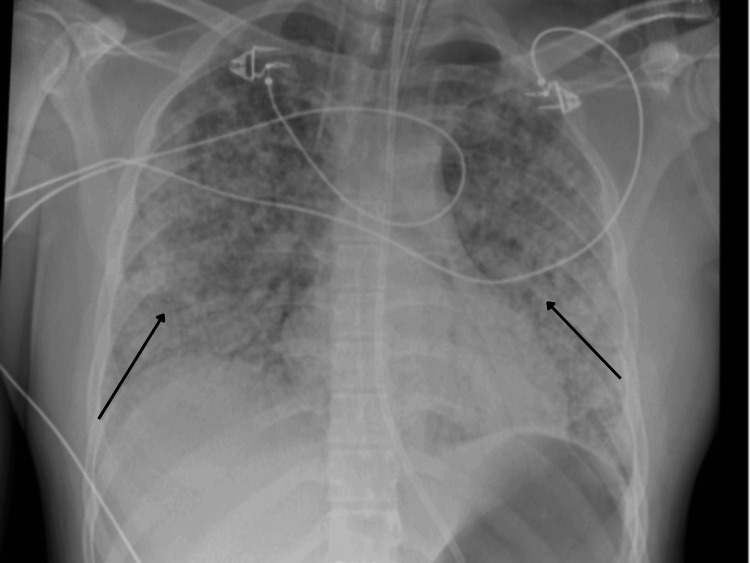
Repeat Chest X-ray four hours from initial Chest X-ray showed worsening bilateral infiltrates
